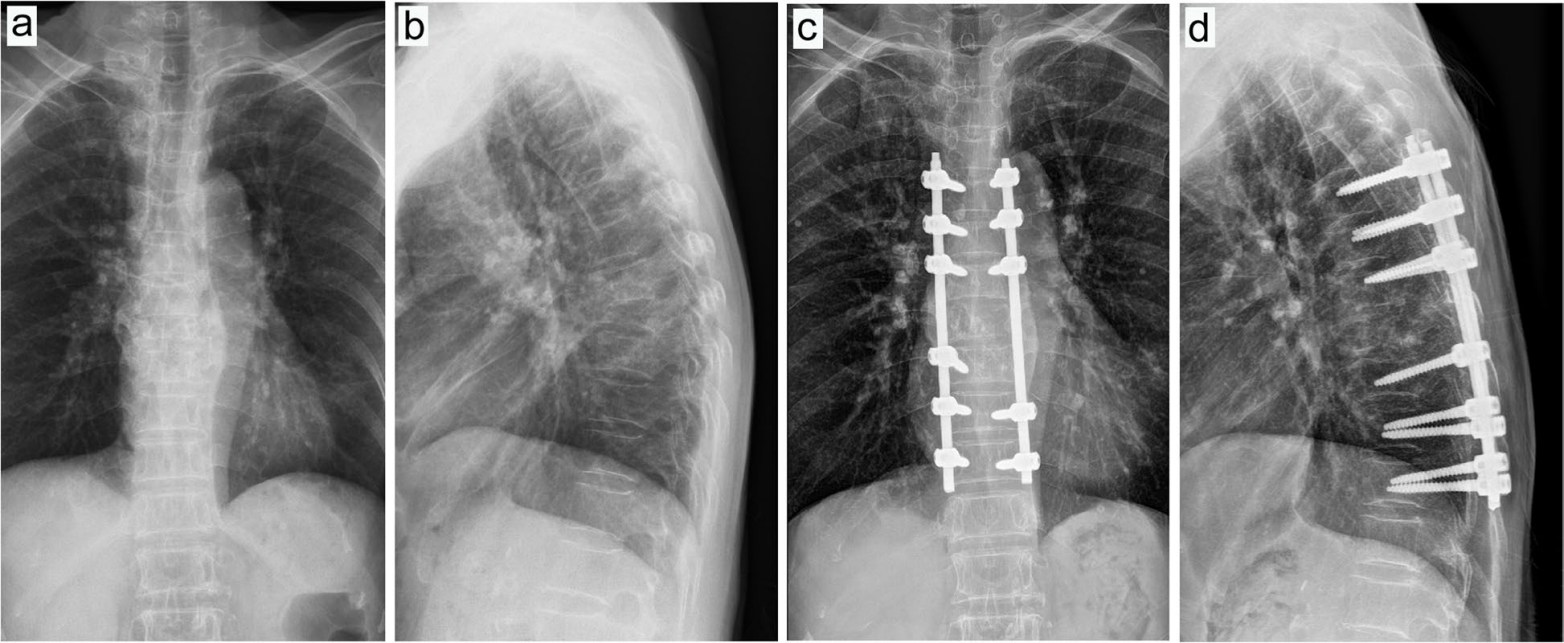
Preoperative and postoperative X-ray examinations in one patient with blood transfusion during surgery. a, Preoperative X-ray examination in the positive position. b, Preoperative X-ray examination in the lateral position. c, Postoperative X-ray examination in the positive position. d, Postoperative X-ray examination in the lateral position
